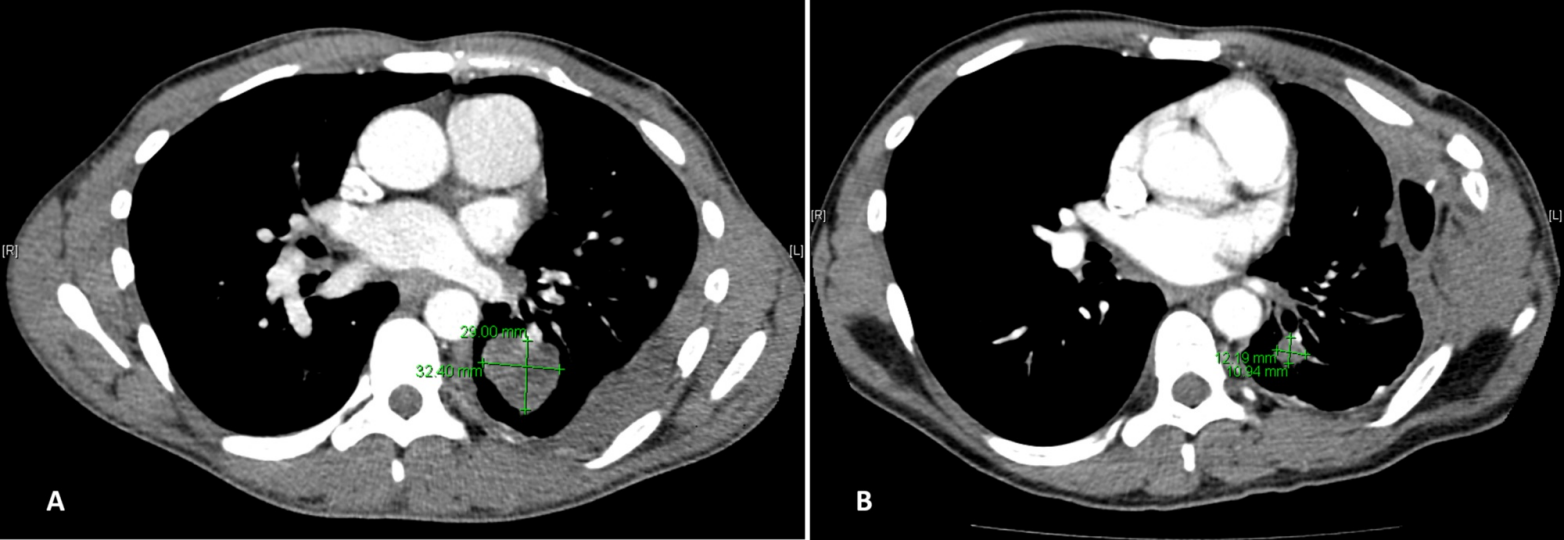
CT scans of the chest in Patient 1 in June 2017 (A) and June 2019 (B).
